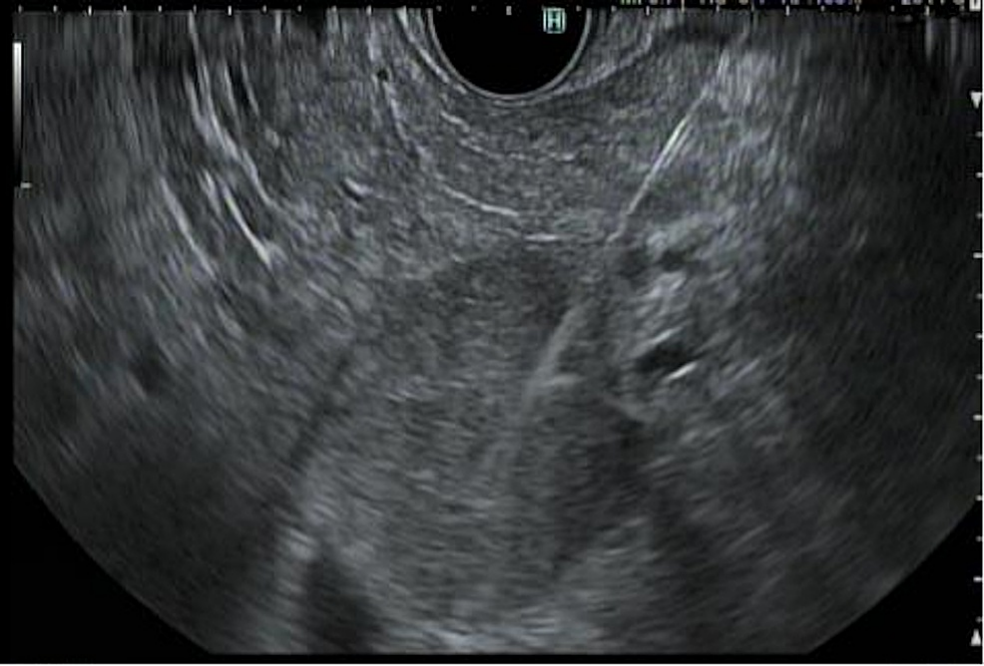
Image of EUS-guided fine-needle biopsy EUS-guided fine-needle biopsy of the pancreatic mass using a 22-gauge needle under Doppler guidance to avoid vascular interference EUS: endoscopic ultrasonography
